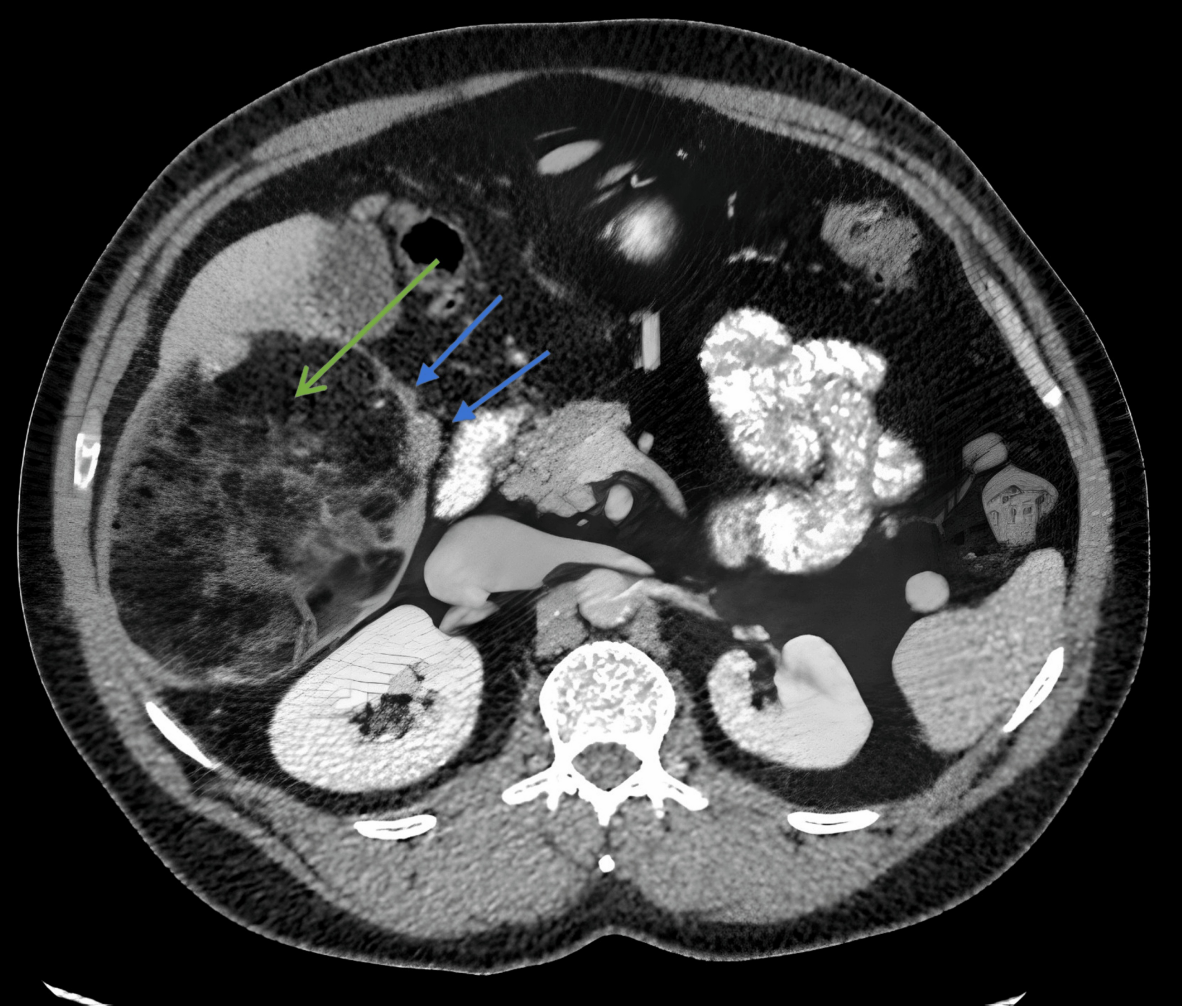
Post-contrast portal venous-phase CT of the abdomen A solitary, large, predominantly fat-density multiseptated lesion arising from and expanding the caudal aspect of the right lobe of the liver (shown in green arrow) with soft tissue mural nodules (shown in blue arrow) CT: computed tomography
